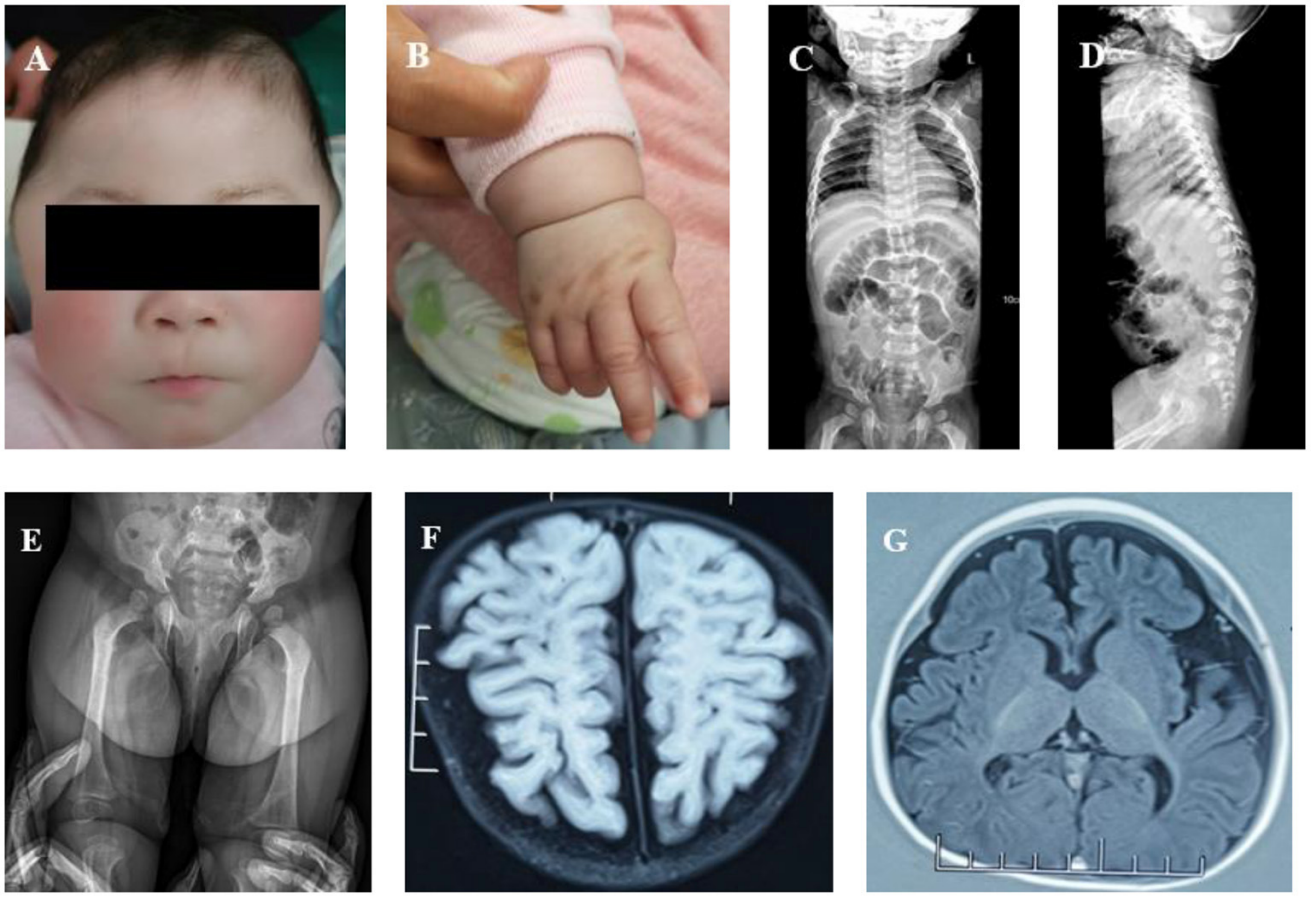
(A) Abnormal facial features (a closed anterior fontanelle, prominent temporal parts, long eyelashes, sparse hair, upturned nostrils, a sharp beak-like mouth, low-set ears, a short neck, and white skin). (B) Wrist joint seam showing significant stenosis. (C) Spine X-ray: slight scoliosis of the spine and slightly narrow vertebral ends of ribs. (D) Spine X-ray: the spine showed slight kyphosis at the junction of the thoracolumbar segment, had short anterior and posterior diameters of some thoracolumbar vertebra, and there was anterior breakage of the vertebral body. (E) Spine X-ray: irregular acetabulum and lower edge of iliac bone. (F) Brain MRI: poor white matter development. (G) Brain MRI: smaller frontal lobe and thinner corpus callosum.
